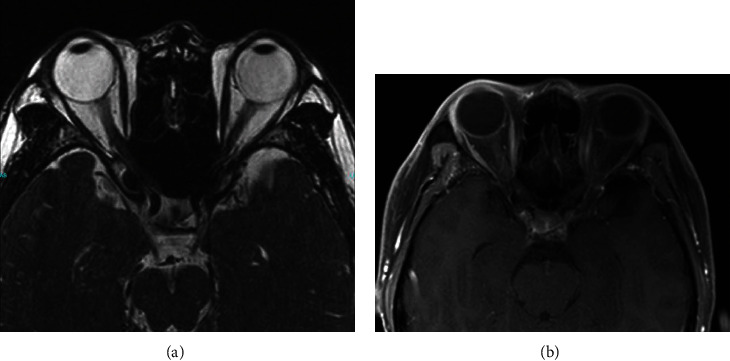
Magnetic resonance imaging (MRI) of head and orbits without evidence of optic nerve abnormality on (a) T2 image and without perineural enhancement of the optic nerve on (b) T1 with contrast and fat suppression.
